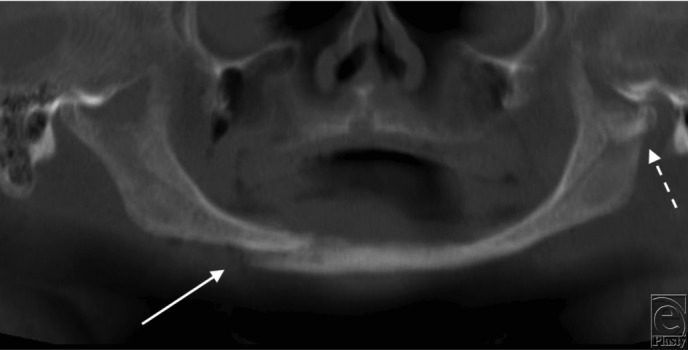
Preoperative panoramic radiographic imaging indicating a right mandibular body (solid arrow) and left subcondylar (dashed arrow) fractures in an edentulous mandible.
